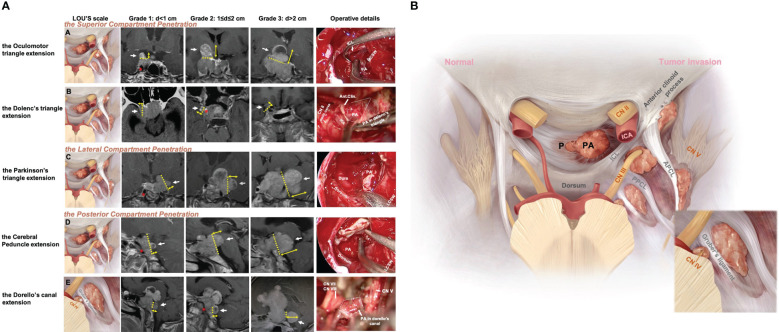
Five patterns of Knosp4PA with cavernous sinus compartment penetration and intracranial extension. (A), Left column, Graphic schemes, the white star indicates penetration region, i.e., extension into (row-A) the oculomotor triangle, (row-B) the Dolenc’s triangle, (row-C) the Parkinson’s triangle, (row-D) the cerebral peduncle, (row-E) and the Dorello’s canal.; Middle column, sellar region MRI images red star indicates encased ICA; Right column, endoscopic and transcranial views. A dashed line indicates penetration entrance. (B), Graphic schemes show the CS penetration pattern (right) compared to the normal anatomical structure of the pituitary(left) located in the sella region. The lower right figure shows a zoomed view of Dorello’s canal penetration. The EXTENSION GRADE was estimated practically by preoperational MRI based on the maximum diameter from the growth entrance, which was delimited superiorly (APCL-PPCL-ICL), laterally (CNIV (trochlear nerve)-CNV1(optical nerve)-PPCL), and posteriorly (PPCL-dorsum-Gruber’s ligament) and was categorized as grade 0 (CS invasion without Penetration), grade 1 (Penetration <1 cm), grade 2 (Penetration =1–2 cm), or grade 3 (penetration >2 cm). APCL, anterior petroclinoidal ligament; CN, cranial nerve; CS, cavernous sinus; ICA, internal carotid artery; ICL, interclinoidal dural ligament; LCSW, lateral CS wall; MCSW, medial CS wall; P, normal pituitary; PA, pituitary adenoma; PPCL, posterior petroclinoidal ligament.
